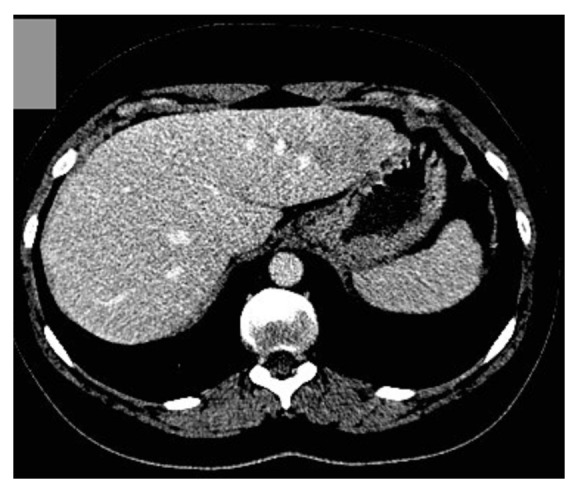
CT scan showing hypovascular lesion in left lobe of liver.
